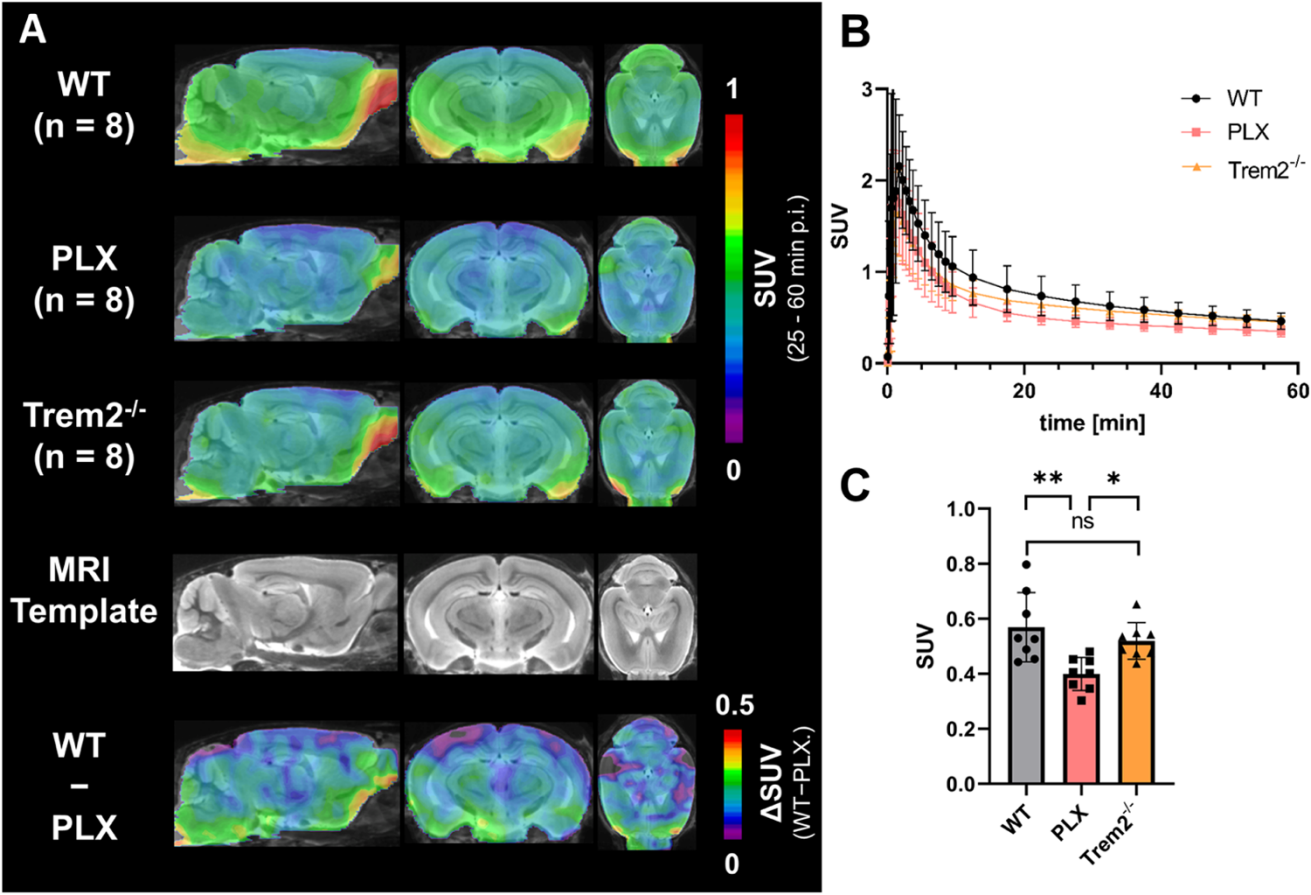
(A) Average PET/CT brain
